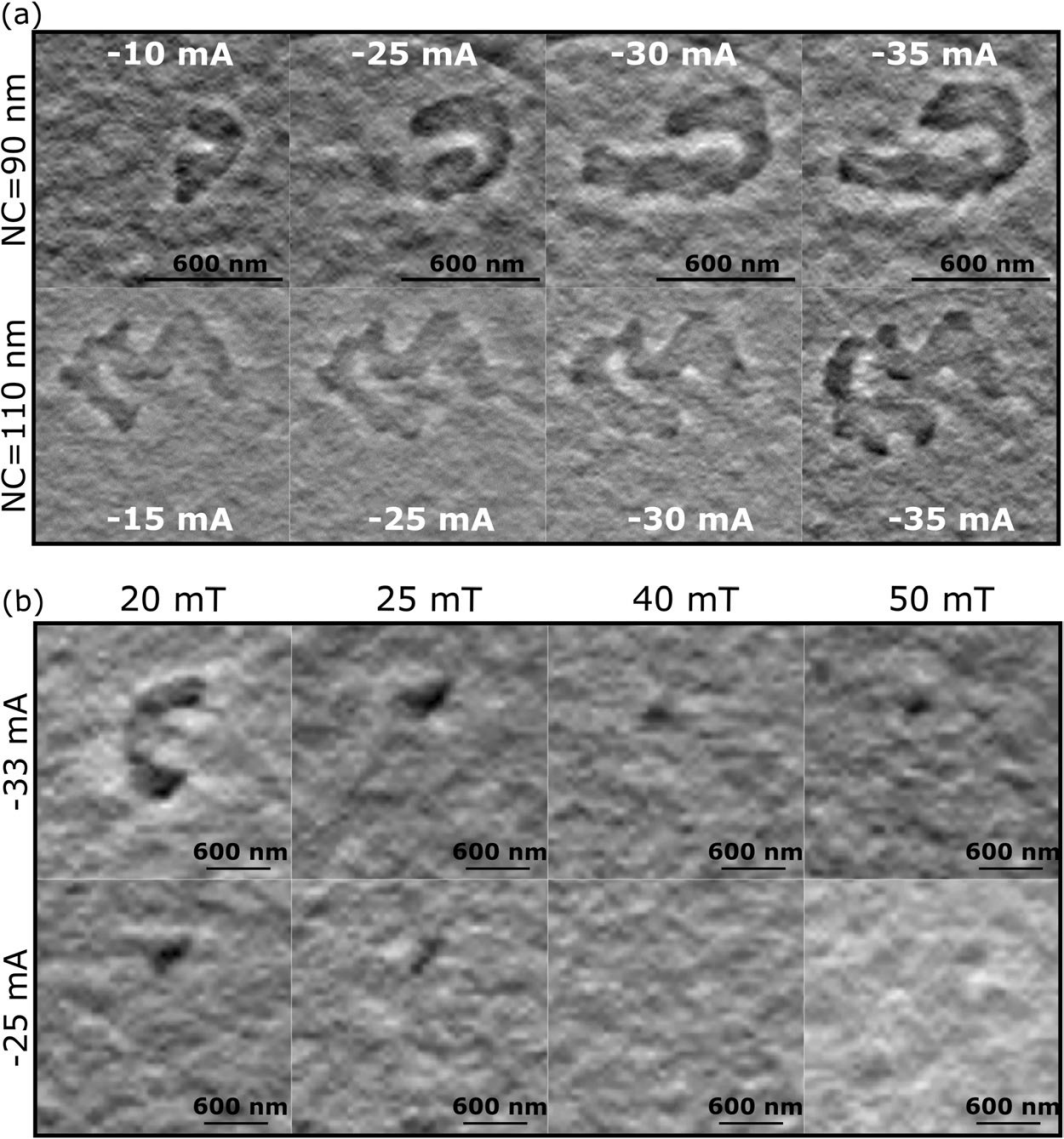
Magnetic structures observed for different fields and applied current values. The samples were saturated by an out-of-plane field of 0.05 T that was then reduced to zero. (a) The darker regions are the magnetic domains created by passing a DC current of −10, −15, −25, −30 and −35 mA through NCs of 90 (upper panel) and 110 nm diameter (lower panel) with zero external magnetic field. The nucleation of the domain is followed by small increments in its size as the amplitude of the DC current is increased. (b) The samples were magnetically saturated at 0.05 T and the field was then removed. The DC current injected through the 110 nm NC was fixed at values of −33 (upper panel) and −25 mA (lower panel) and HERALDO measurements were made with fields of 20, 25, 40 and 50 mT applied perpendicular to the sample plane in the direction of the initial saturation, parallel to the direction of the x-rays. The domain size decreases as the external magnetic field is increased, vanishing when the external magnetic field is sufficient to overcome the influence of the Oersted field produced by the current.
